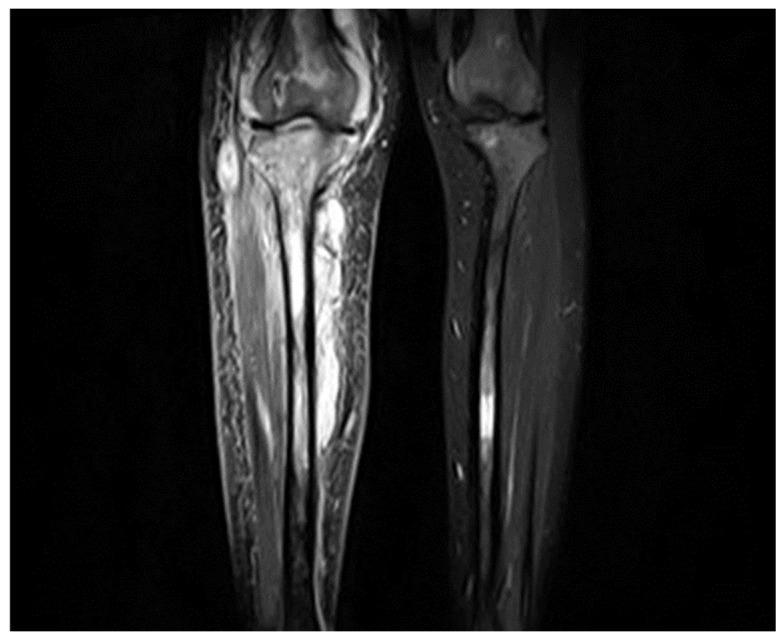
Coronal T1-weighted MR image of a 26-year-old female with fluid collection surrounding the tibia with underlying bone changes indicating tibial osteomyelitis.
